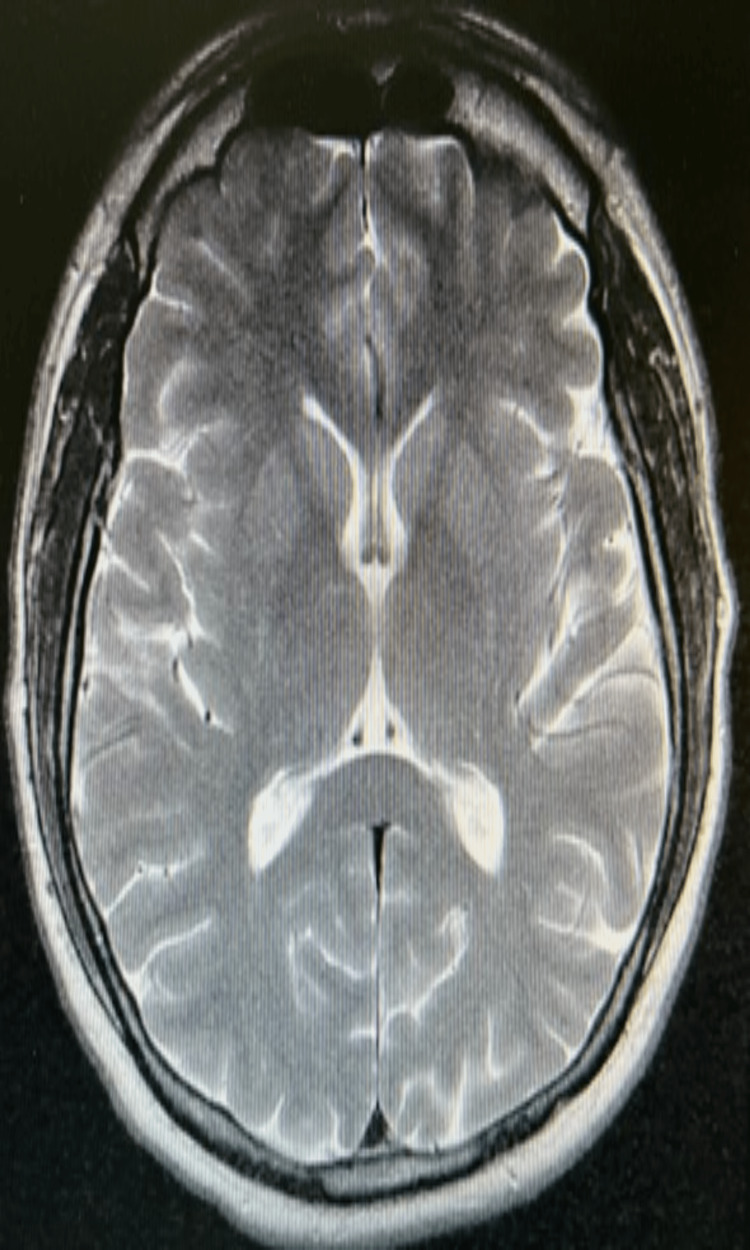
MRI of the brain was negative for thrombus and hemorrhage
